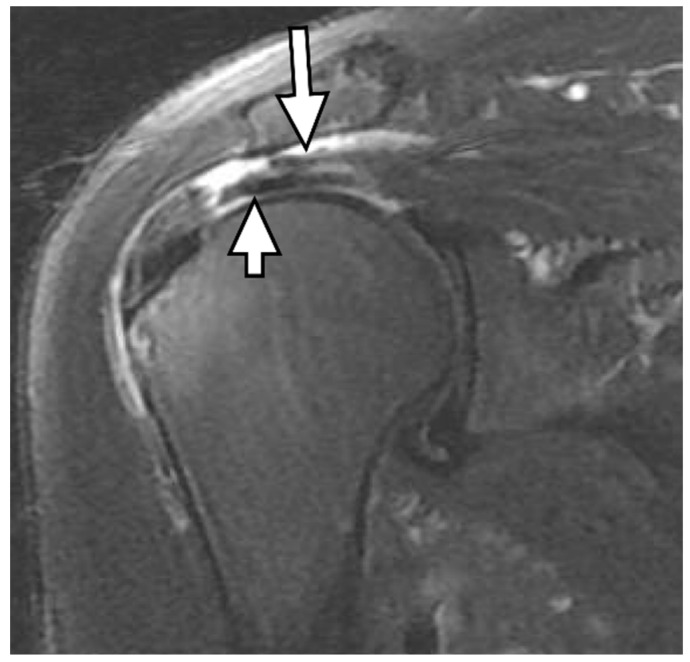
Bursal-delaminated full-thickness tear of the supraspinatus tendon. An oblique coronal fat-suppressed T2-weighted image of the supraspinatus tendon shows a bursal-delaminated full-thickness tear with a visible cleavage. The bursal surface (long arrow) is retracted more medially than the articular surface (short arrow).
